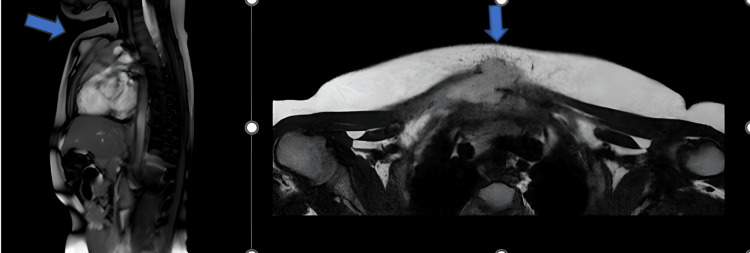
Appearance of a mass extending to the subcutaneous tissue in the midline of the retrosternal region in the thoracic MRI taken at the patient's first admission
